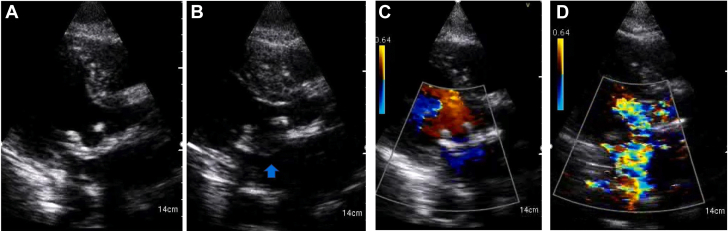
POCUS images showing bioprosthetic mitral valve in diastole (A), flail leaflet of mitral valve prothesis (*blue arrow* in panel B), color Doppler image diastole (C), and severe MR on color Doppler imaging (D) during systole.
